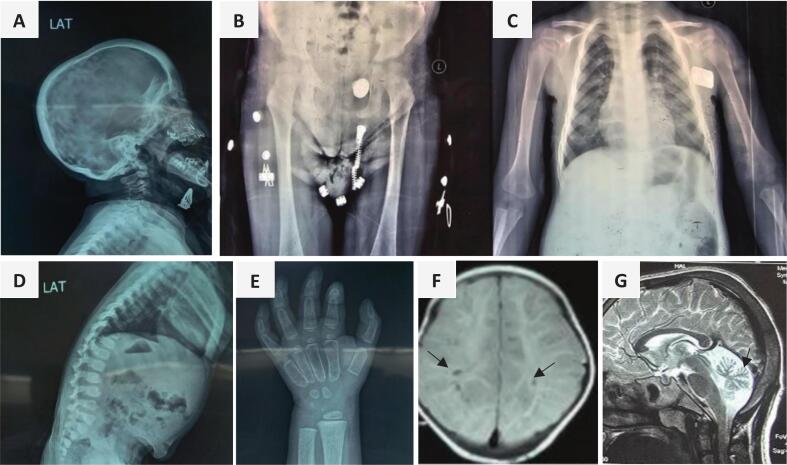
(A–G): Imaging findings in patients with alpha-mannosidosis: A–E, (Dysostosis multiplex): A) P5 - macrocephaly and calvarial thickening (beaten copper is also manifested); B) P4 - Underdeveloped acetabulum with rounded iliac crest; C) P4 - posteriorly widened ribs; D) P5 - ovoid (anterior beaking) vertebral bodies and gibbus; E) P5 - hand shows bullet-shaped phalanges; F) P4 - MRI findings (T1WI), Prominent Virchow – Robin spaces (black arrows); G) P9 - MRI brain, (T2WI sagittal), cerebellar atrophy.
